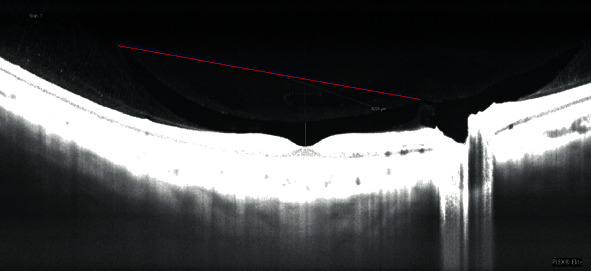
Width (W) and central thickness (CT) of bursa premacularis on longitudinal OCT scans. W was assessed considering a line combining the most distant points from foveal depression. CT was considered from foveal depression to superior border of bursa.
